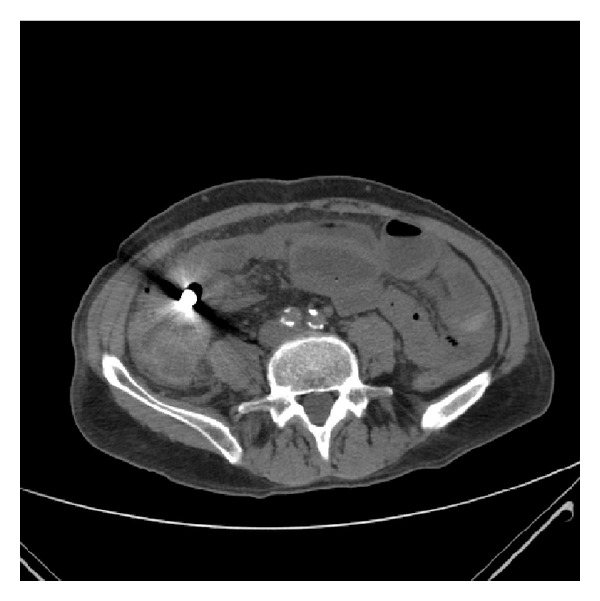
CT-abdomen showing small bowel obstruction, radiodense foreign body (capsule endoscope), and intraperitoneal air.
